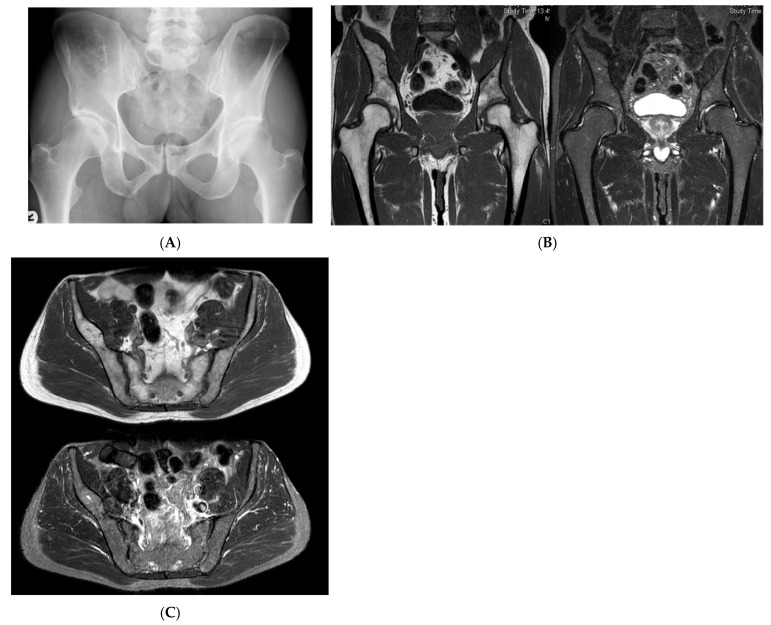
Intraosseous lipoma, right iliac bone. (A) AP radiograph demonstrating a right iliac bone lesion. (B) Coronal TW1 and STIR MRI side by side, demonstrating a right iliac fat-containing bone lesion exhibiting fat suppression. (C) Axial T1W MRI showing a right iliac bone lesion and axial STIR MRI of the same lesion with fat suppression.
